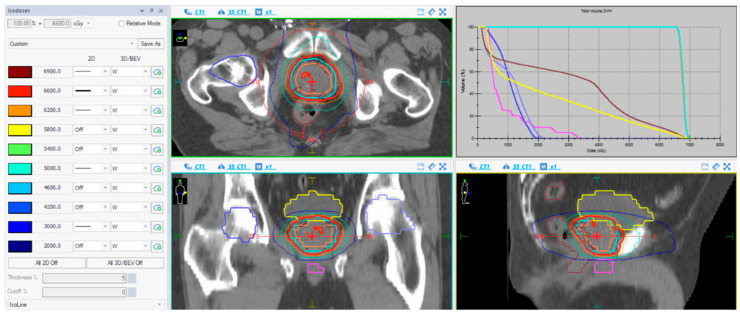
Dose-volume histogram (upper-right image); a blue line represents the PTV coverage, brown and yellow lines represent the dose received by the rectum and bladder, respectively. CT images of volumes and isodose distribution of a VMAT plan in the axial, coronal and sagittal planes (the rest of images); a blue line represents PTV margin. Treatment plan showing the radiation dose distribution in colored, lines within the interval from 30 Gy (dark blue) to 66 Gy (red).
